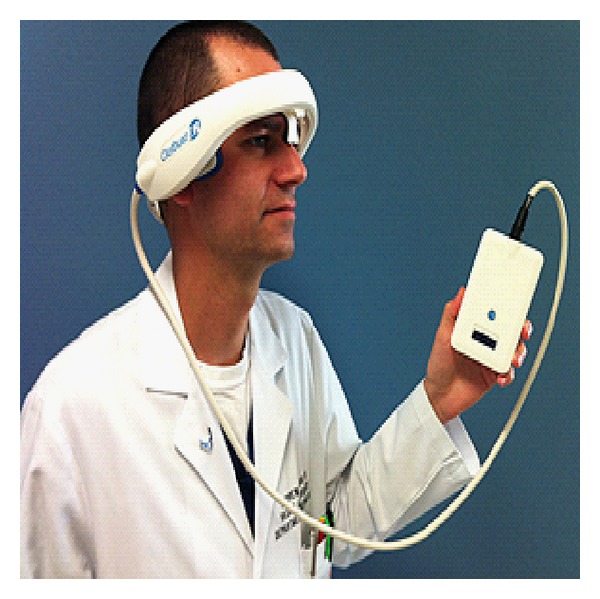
The Clotbust ER ultrasound system for thrombolysis. Courtesy of Cerevest Therapeutics Inc.
